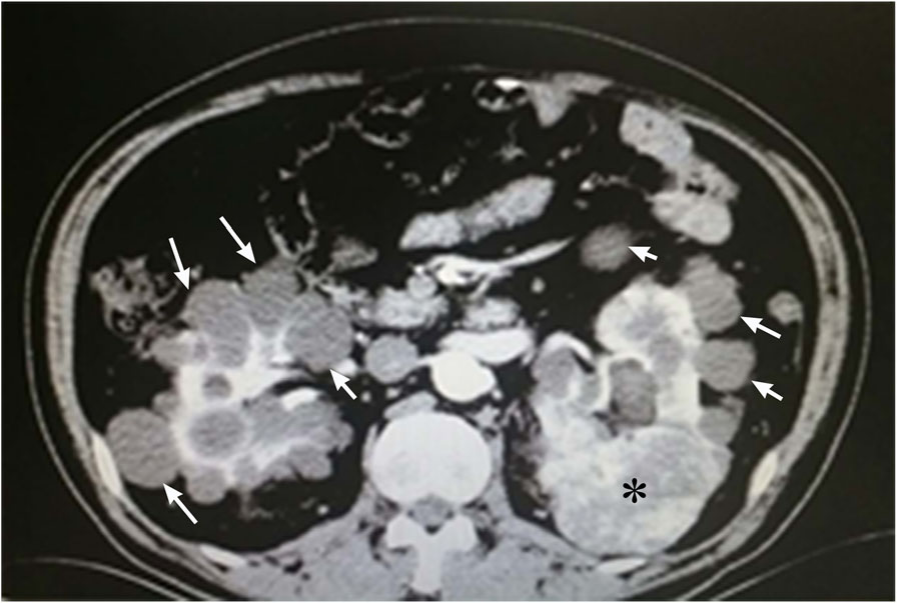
CT image of bilateral kidney tumours and polycystic kidneys. CT images were taken from both kidneys at the lumbar level from the subject of case 1. Arrows indicate multiple kidney cysts. Asterisks indicate kidney tumours
